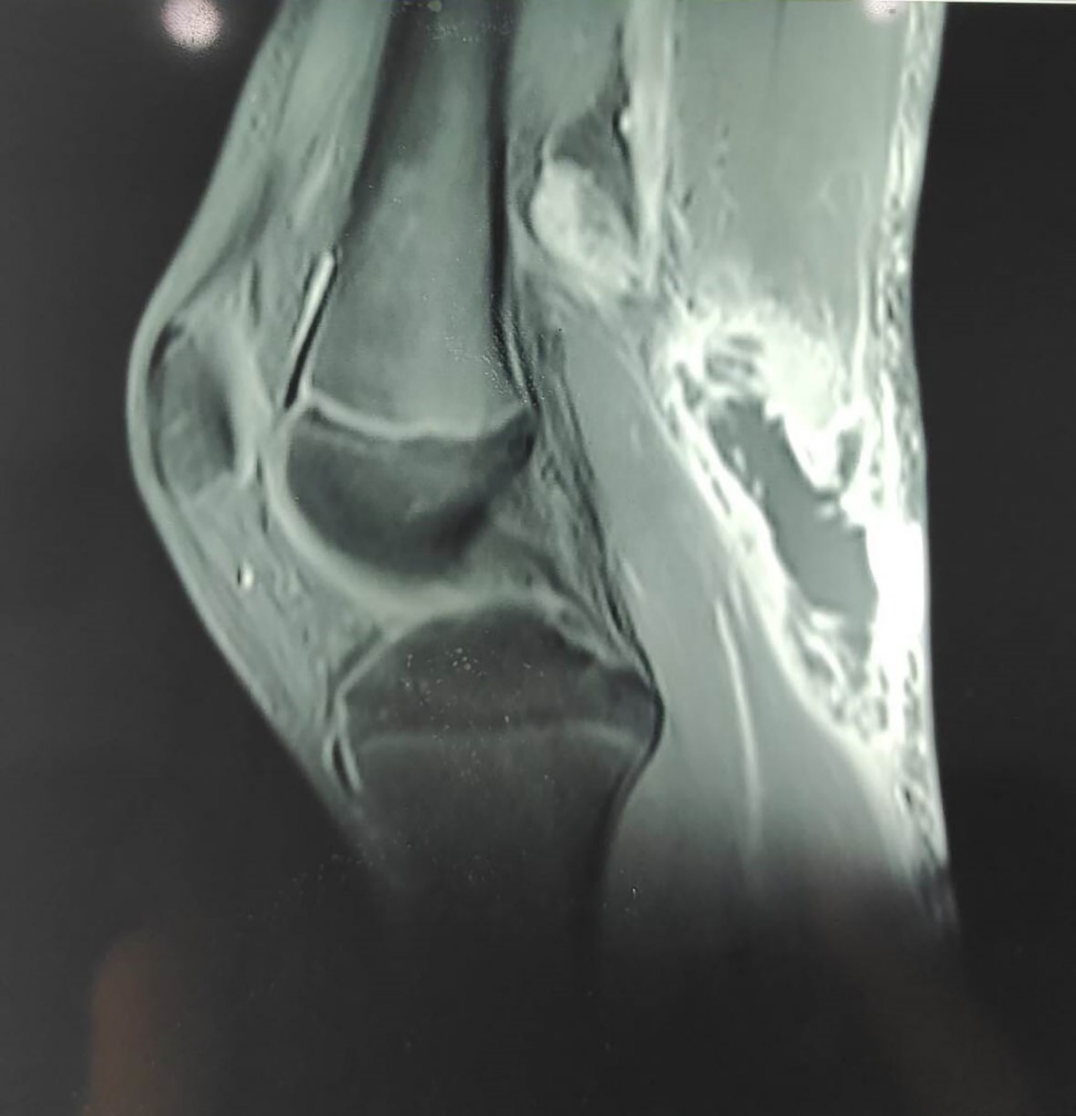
T1-weighted MRI reveals a vascularized mass adjacent to the semimembranosus and medial gastrocnemius muscles.
